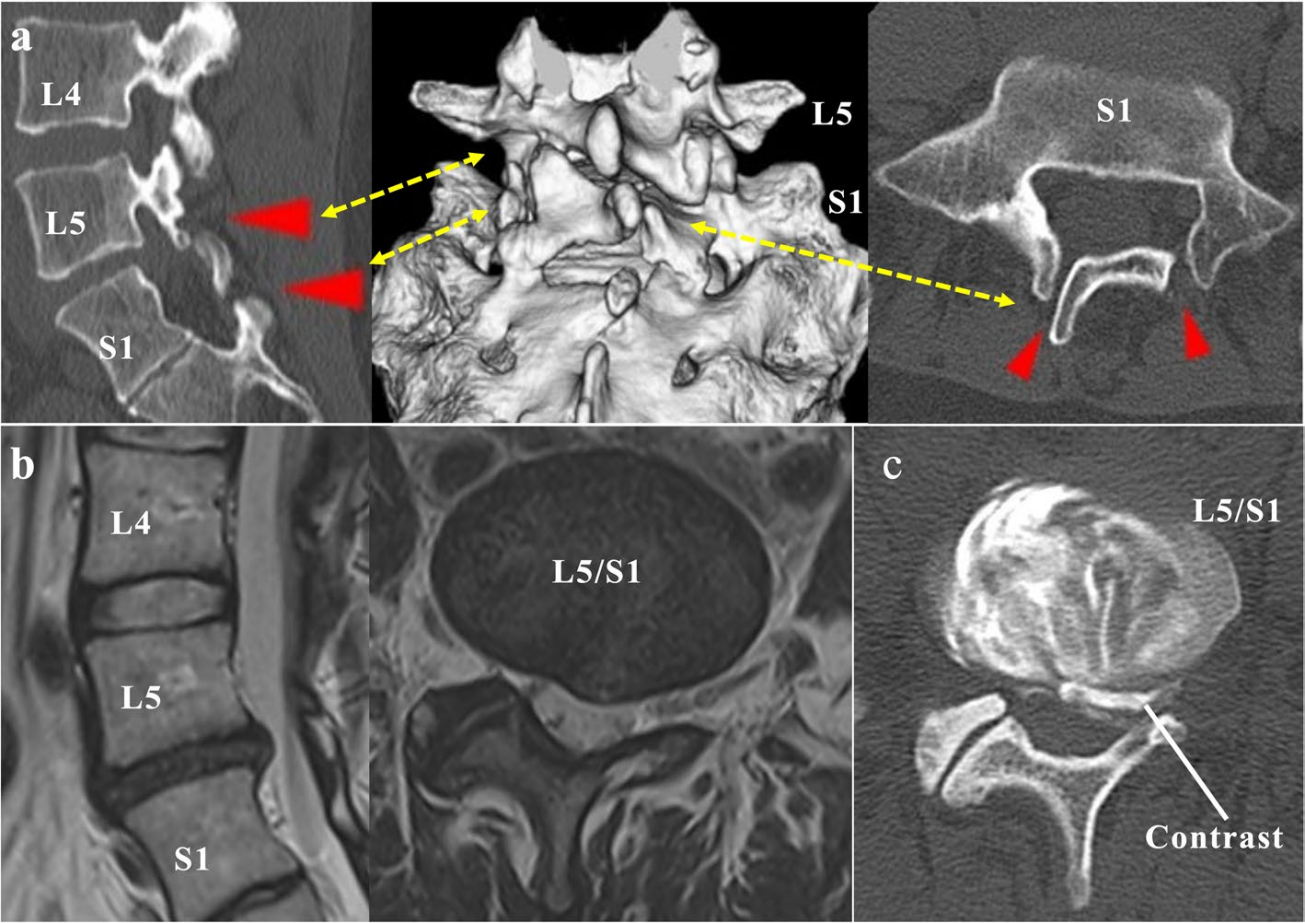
Preoperative image evaluation. a Sagittal, axial, and reconstructed 3-dimensional computed tomography images show the left L5/S1 abnormal facet complex and unco-ossified S1 lamina (red arrows). b Sagittal and axial T2-weighted magnetic resonance imaging show the L5/S1 degenerative disc compressing the existing left S1 nerve. c Axial computed tomography discography shows the morphologically violated disc at L5/S1. The contrast medium enters the posterior annulus
